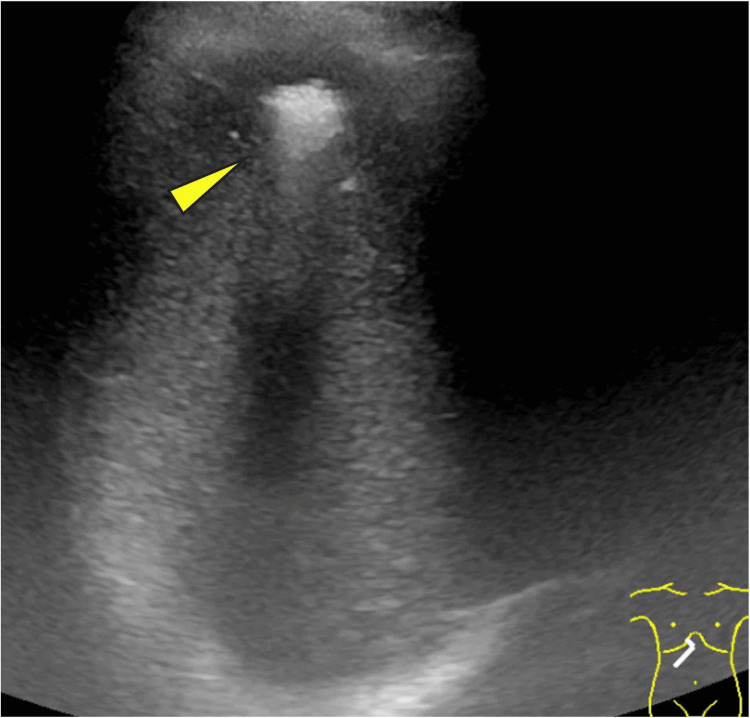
Abdominal ultrasound Abdominal ultrasound demonstrated a hyperechoic area suggestive of emphysematous change with no evidence of abscess formation (arrowhead).
